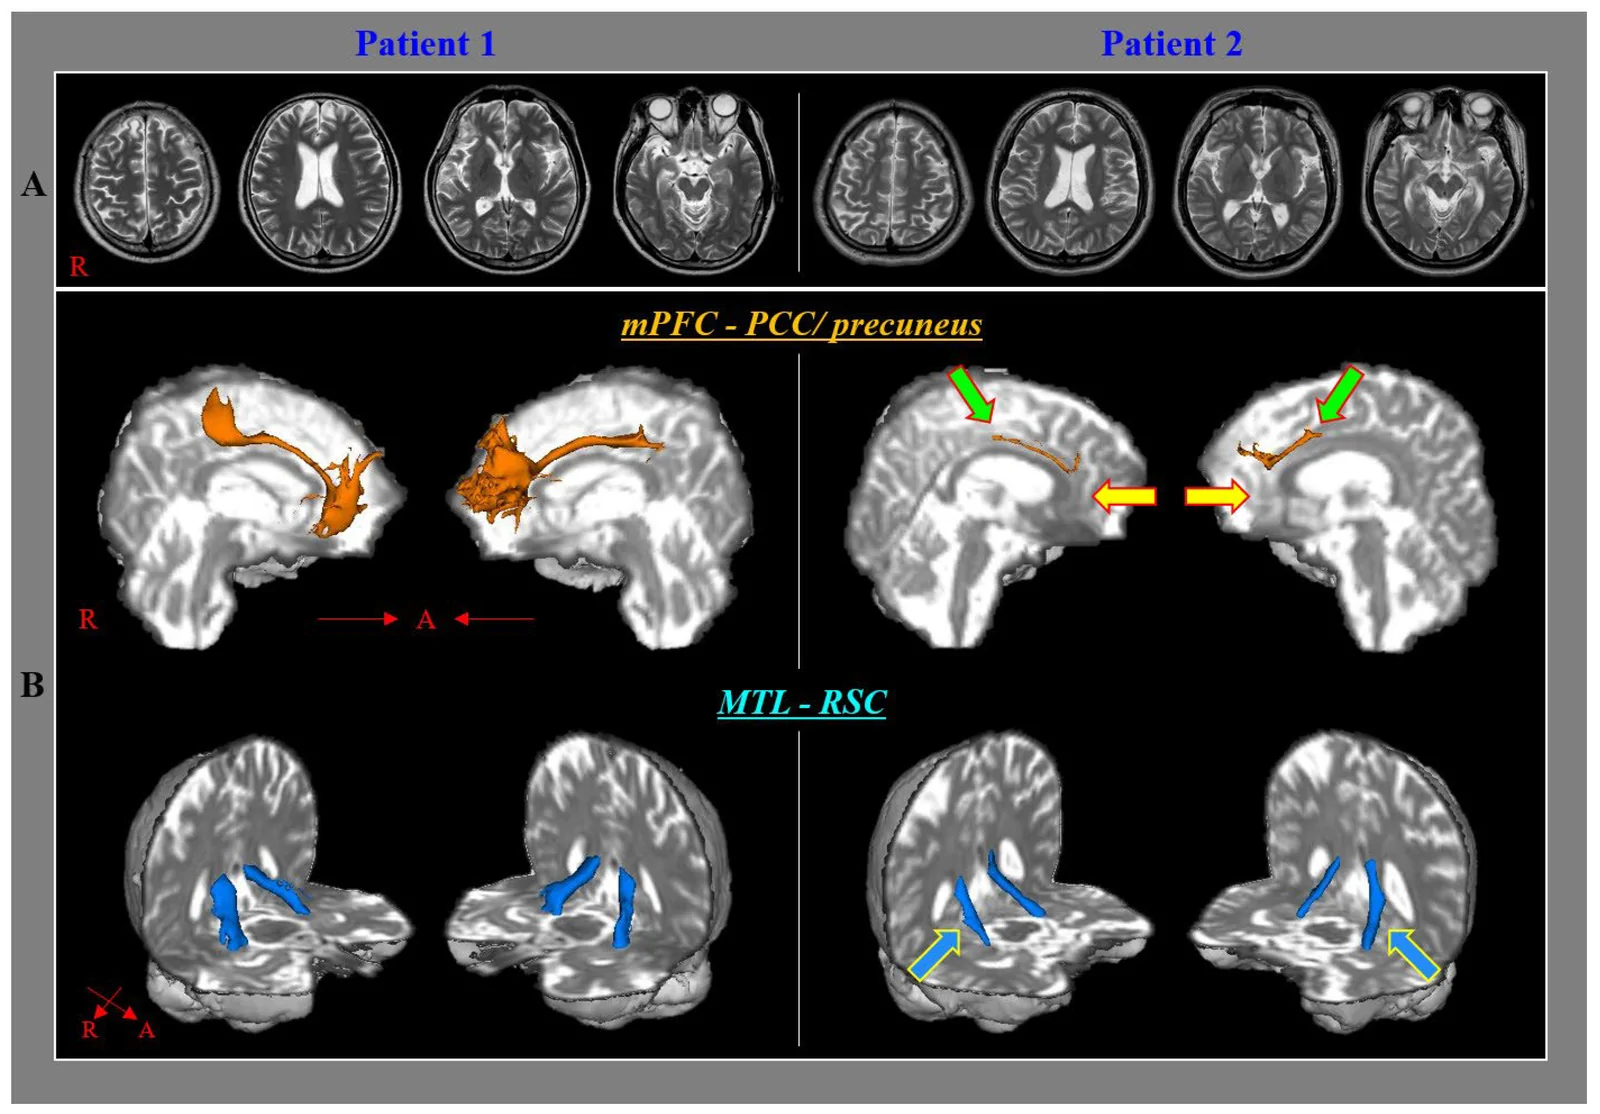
Results of diffusion tensor tractography for the default mode network (DMN) connectivity [medial prefrontal cortex (mPFC)—posterior cingulate cortex (PCC)/precuneus, medial temporal lobe (MTL)—retrosplenial cortex (RSC)]. (A) T2-weighted brain magnetic resonance images at the time of diffusion tensor imaging scanning in representative patients with a diffuse axonal injury at the chronic stage [patient 1: 45-year-old male with four days of loss of consciousness (LOC) duration, patient 2: 23-year-old male with 30 days of LOC duration]. (B) The mPFC—PCC/precuneus neural connectivity in patient 2 shows discontinuation near the mPFC and PCC/precuneus (green and yellow arrows). In addition, narrowing fibers of the MTL—RSC neural connectivity in patient 2 are reconstructed (sky blue arrow) compared with those in patient 1.
